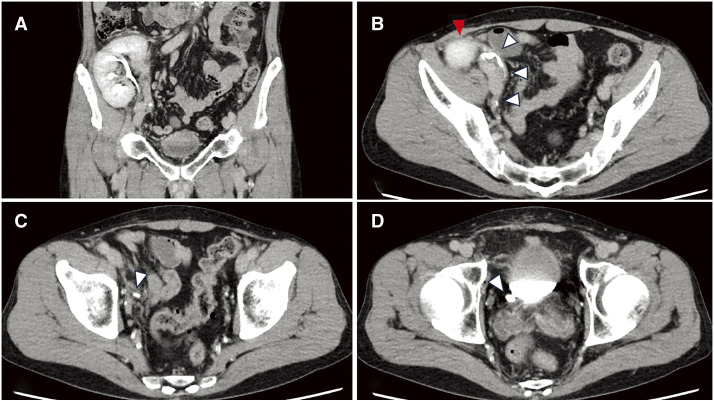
CE-CT findings. (A) The transplanted kidney is located in front of the iliac crest (coronal view). (B–D) The ureter (white arrowhead) runs medially, dorsally, and caudally from the transplanted kidney (red arrowhead) and enters the bladder (axial view). CE-CT, contrast enhanced CT
